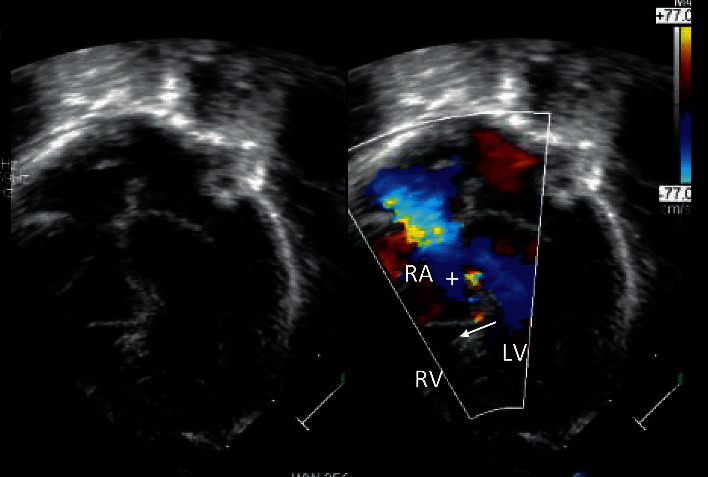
Transthoracic echocardiogram with 2D and color Doppler showing an apical displacement of septal leaflet of the tricuspid valve (arrow) and atrialization of RV. A left to right shunt is also seen across a VSD into the atrialized RV.
